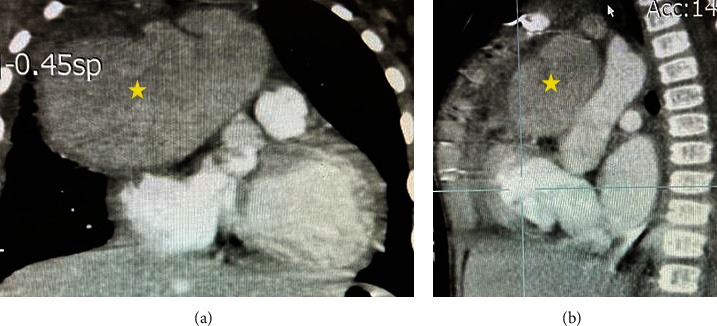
Coronal (a) and sagittal (b) views of contrast enhancement CT image show a large pseudoaneurysm arising from the ascending aorta (asterisks).
